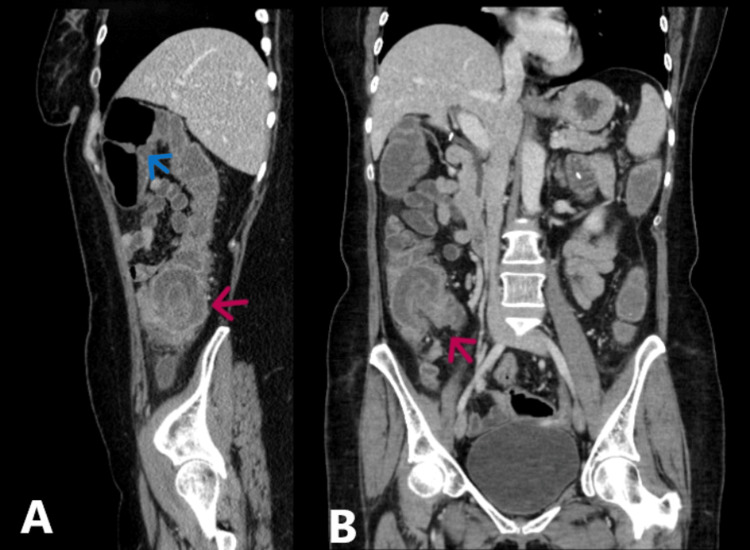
CT abdomen and pelvis demonstrating circumferential thickening within the mid-transverse colon (blue arrow) and features of telescoping/ileocaecal intussusception of the terminal ileum onto the caecum (red arrows) CT: Computed tomography
